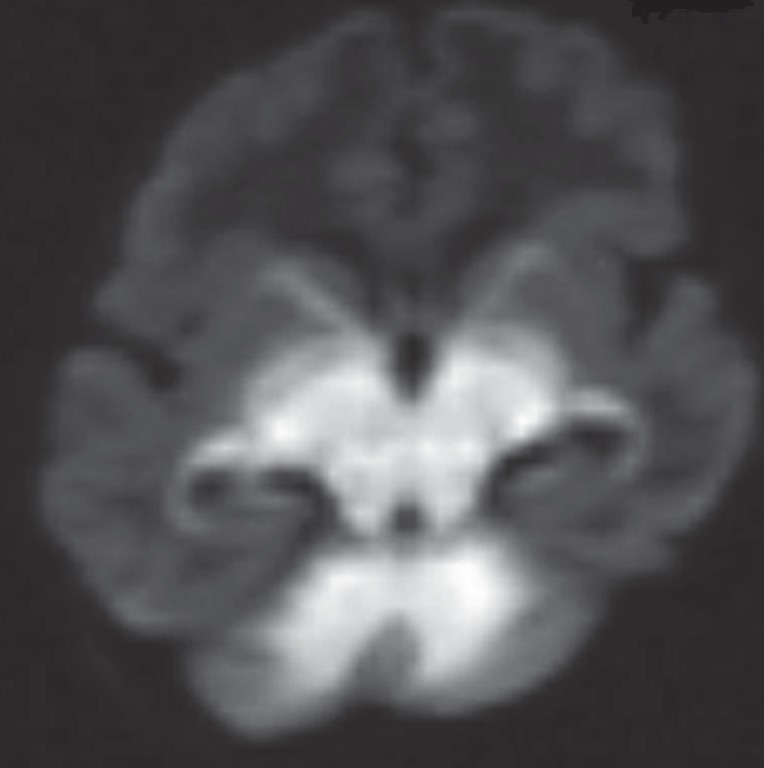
DWI shows hyperintensity in the myelinated white matter areas
